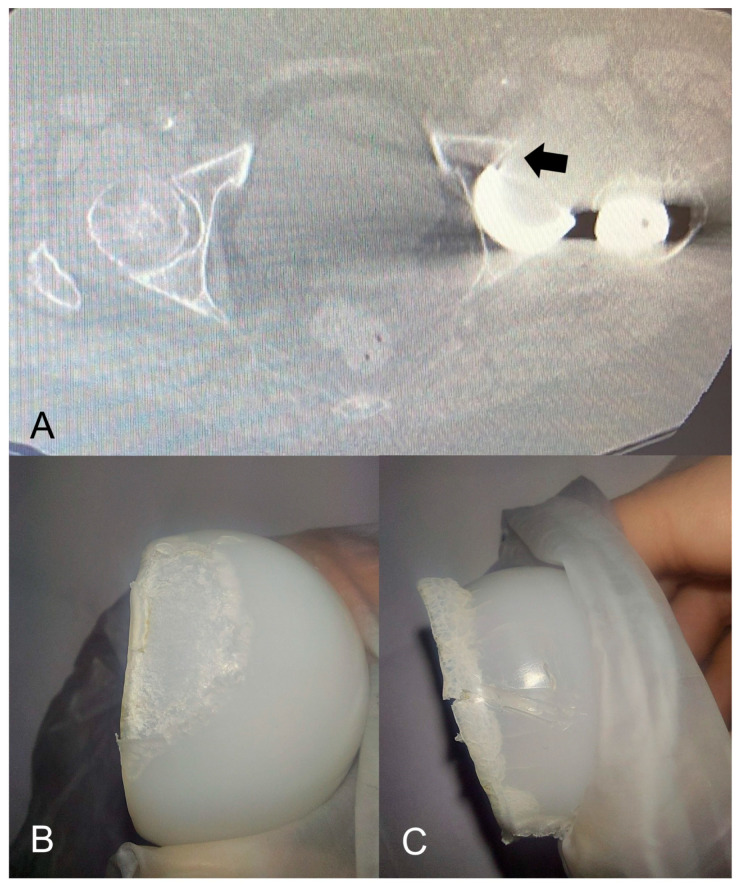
IPD case occurred 41 months after modular DM THA. (A): CT scan image showing the acetabular cup implanted with 55° of anteversion, leaving the anterior portion of the acetabular cavity uncovered (black arrow). (B,C): Massive wear and deformation of the mobile polyethylene liner were observed at revision along the liner edge. These signs on the retrieved component could represent the catastrophic results of a mobile liner impingement against the anterior portion of the acetabulum caused by cup malpositioning for excessive anteversion.
